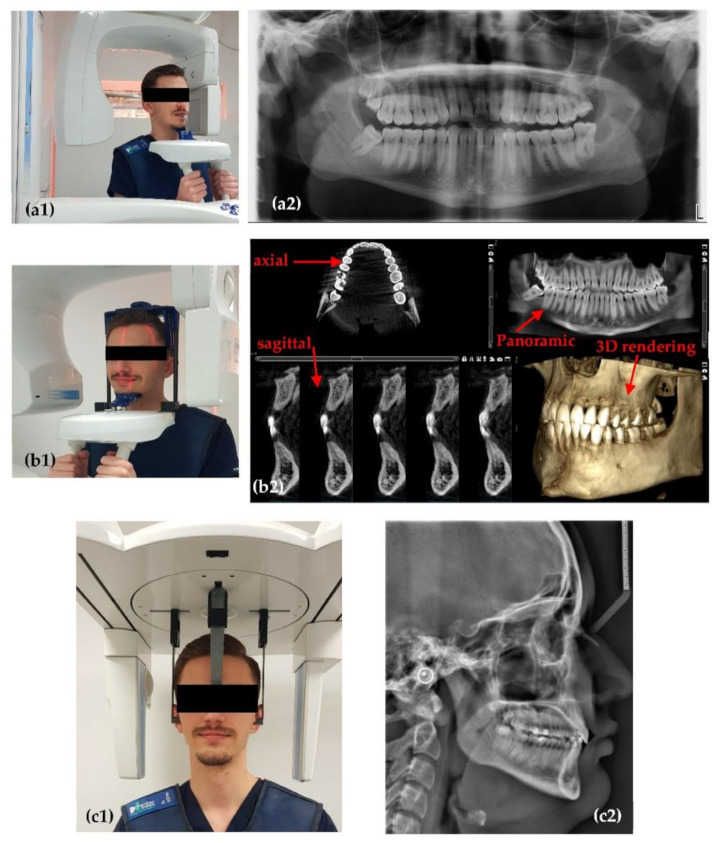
Example of the Planmeca setup for (a1) panoramic, (b1) 3D CBCT, and (c1) cephalometric investigations (of a healthy member of the staff). Obtained images: (a2) panoramic; (b2) 3D CBCT images, with axial, sagittal, and panoramic views, as well as a 3D reconstruction; (c2) cephalometric.
